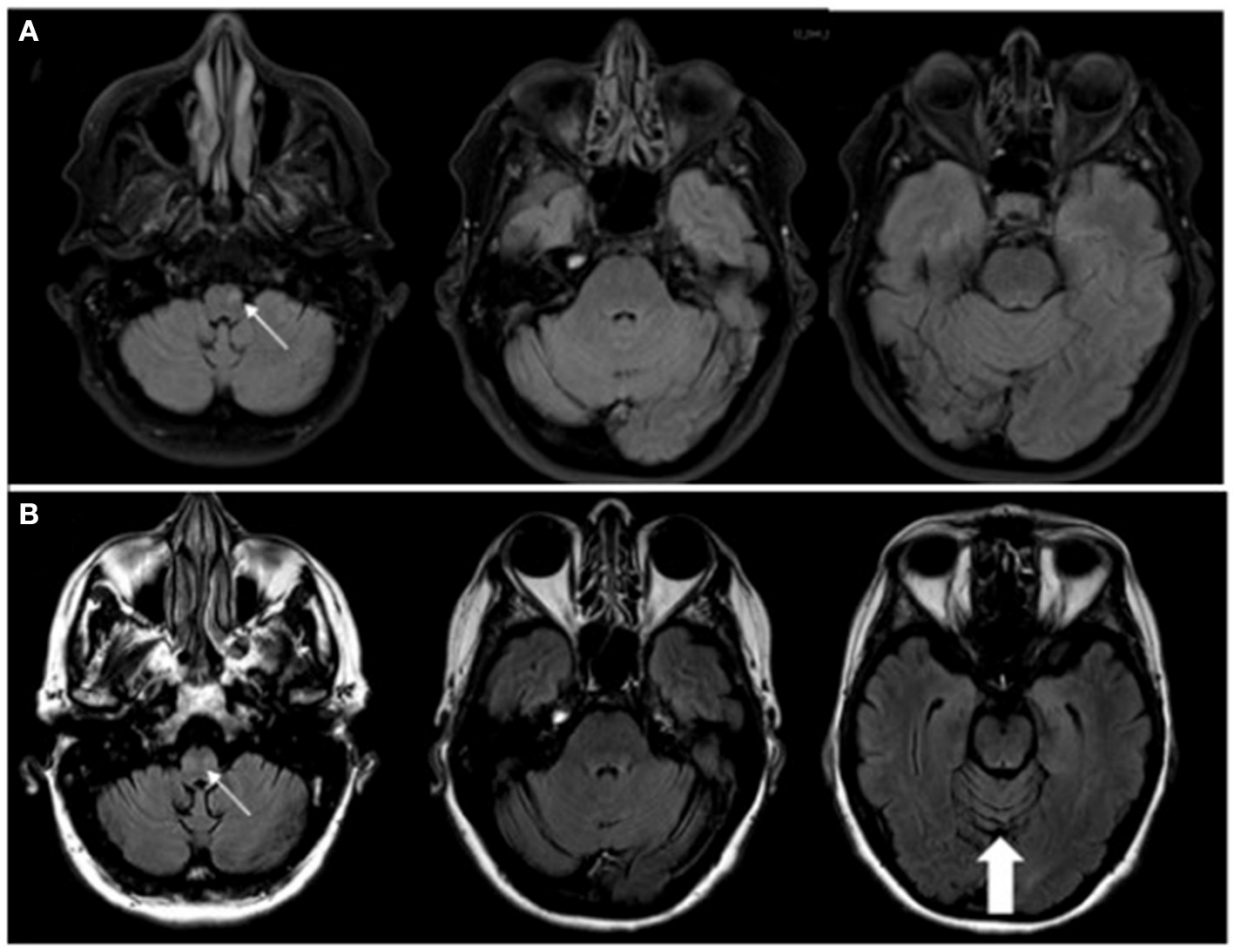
(A) MRI sequences of T2/FLAIR (fluid-attenuated inversion recovery) with STIR (short tau inversion recovery; i.e. fat suppression) at the onset of neurological symptoms of vertigo and ataxia demonstrating a small T2, non-enhancing lesion (contrast imaging not shown) in the left medulla (thin, white arrow). (B) with T2/FLAIR MRI 3 months later demonstrating T2 changes seen bilaterally within the medulla (thin, white arrow) with no associated contrast enhancement. There is noted cerebellar atrophy, particularly seen in the superior cerebellum (thick, white arrow), compared to the MRI from 3 months prior.
